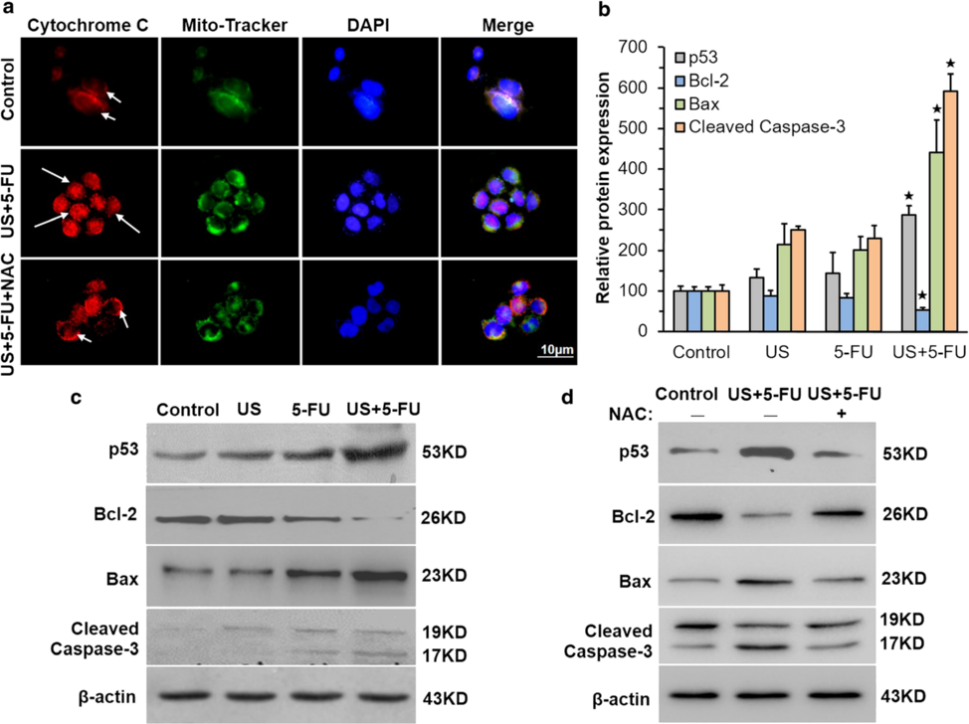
Activation of mitochondrial-caspase pathway of apoptosis by low-intensity ultrasound combined with 5-FU. a Representative fluorescence images of the translocation of cytochrome c in BEL-7402 cells. Cytochrome c in NAC-untreated US + 5-FU group diffused in the cytosol (long arrow), which was anchored to the mitochondria in the Control and NAC-treated US + 5-FU groups (short arrow). Representative expression of apoptosis-related proteins in vivo (b) and in vitro (c) after the treatment. d Representative expression of apoptosis-related proteins with and without NAC in BEL-7402 cells. Data was presented as the mean ± SD (*n* = 6, ^★^
